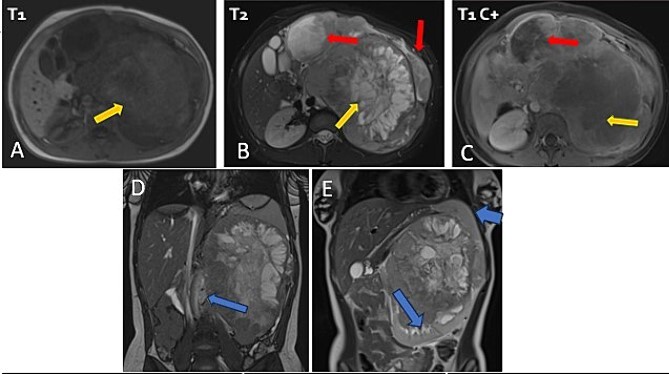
Abdominal MRI in axial T1 (A), T2 (B), and a T1 fat-saturated post contrast (Gadolinium) sequence (C), coronal T2 (D and E) showing a large retroperitoneal tumour process lateralized to the left, hypointense on T1-weighted, hyperintense, and heterogenous on T2-weighted images with central areas of necrosis and contrast-enhancing after Gadolinium administration. Lymph nodes and peritoneal carcinomatosis nodules are associated. Displacement of the vascular axes (D) and of the spleen of the left kidney responsible for left pelvicalyceal dilation (E).
